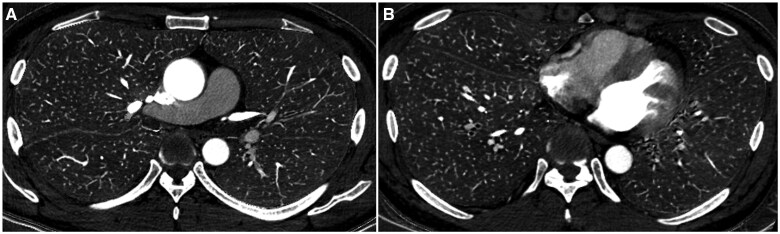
Iodine maps (upper zone [A] and lower zone [B]) of a 48-year-old male patient with a body weight of 71 kg in whom transient interruption of contrast was seen. This patient underwent CT pulmonary angiography at the end-inspiratory level. A contrast agent with an iodine concentration of 370 mgI/mL was injected from the right antecubital vein. The iodine concentration of the right upper, right middle, right lower, left upper and left lower lobes, and main pulmonary artery was 0.69, 0.52, 0.45, 0.52, 0.58, and 3.9 mgI/mL, respectively.
